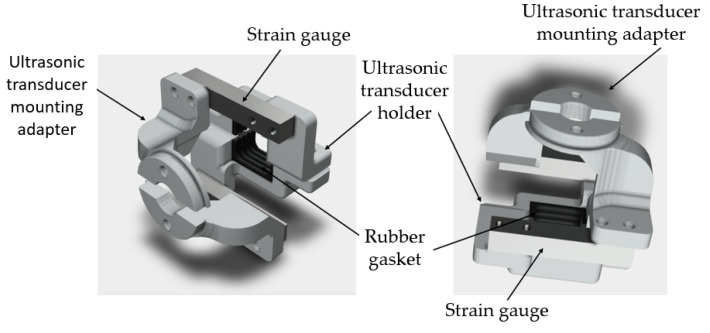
Design of attachment of the ultrasonic sensor and strain gauge.
